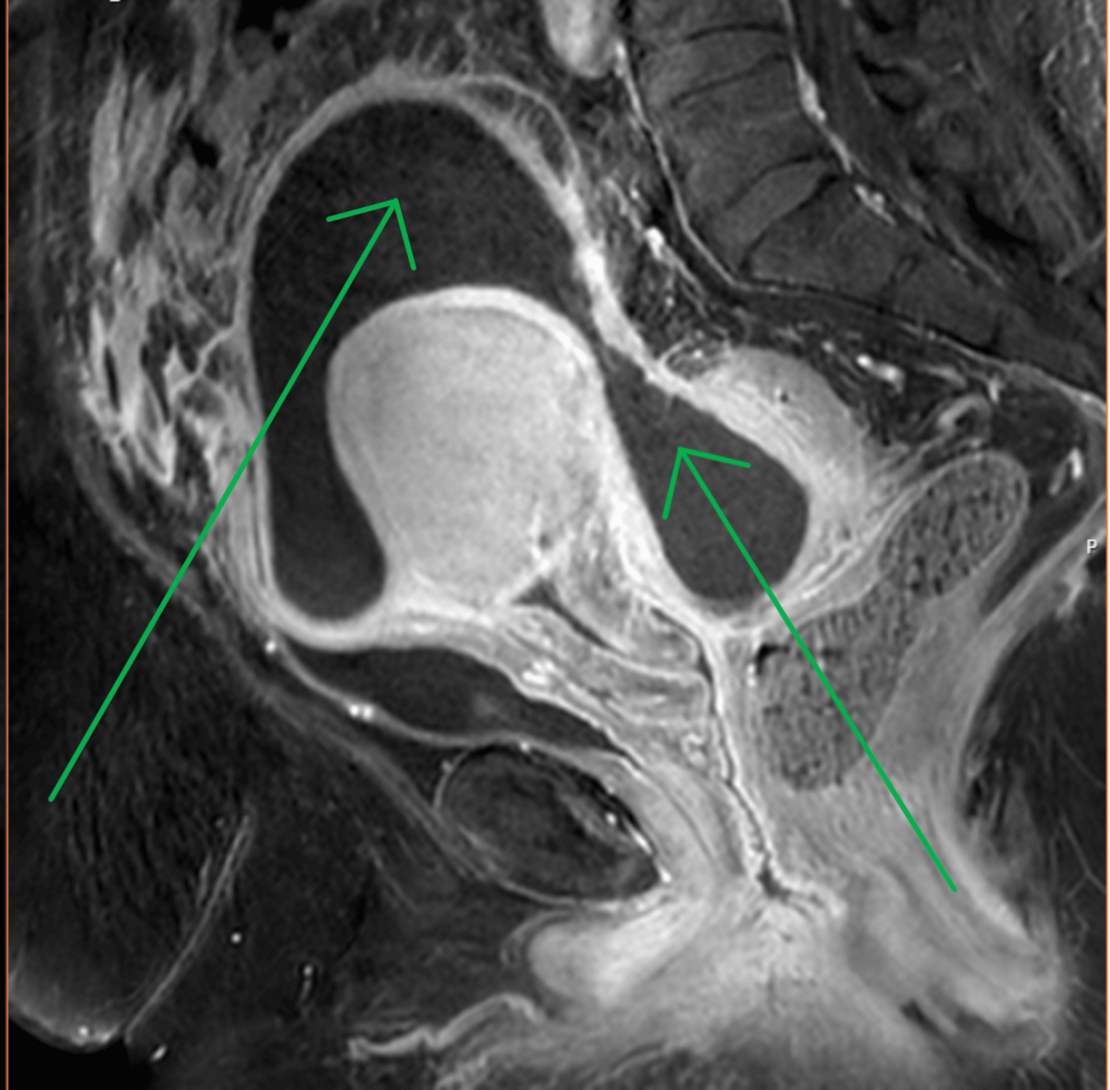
MRI demonstrating a superior and anterior collection to the uterine fundus and a smaller collection in the rectouterine space
